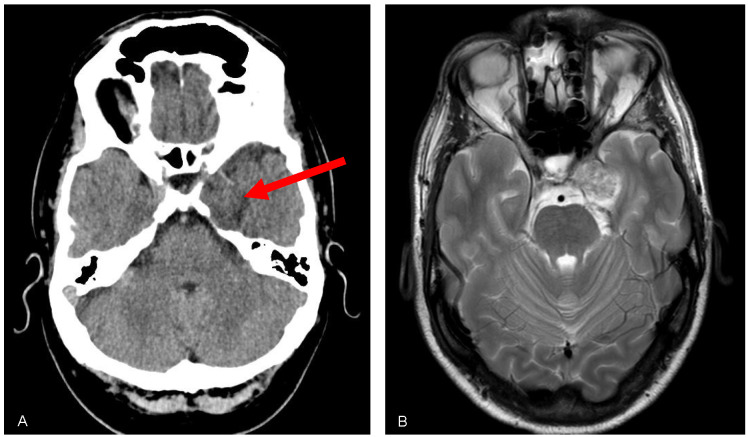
A: Note the lesion on CT scan (red arrow), which remodels the adjacent bone structures. B: MRI shows a nodular T2 enhancing mass in the region corresponding to the trigeminal fossa.
